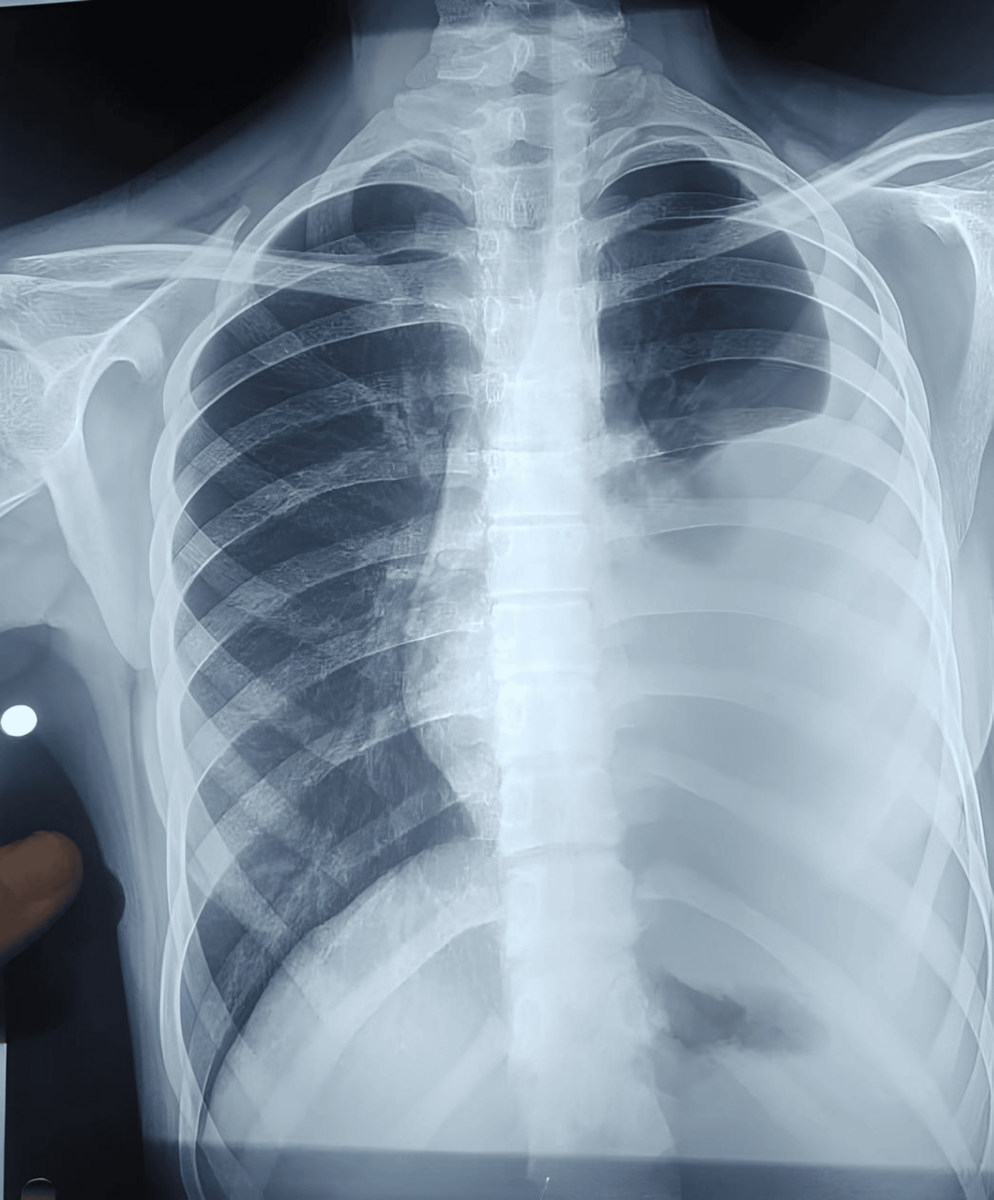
Chest X-ray showing left sided pleural effusion (pre-thoracocentesis)
